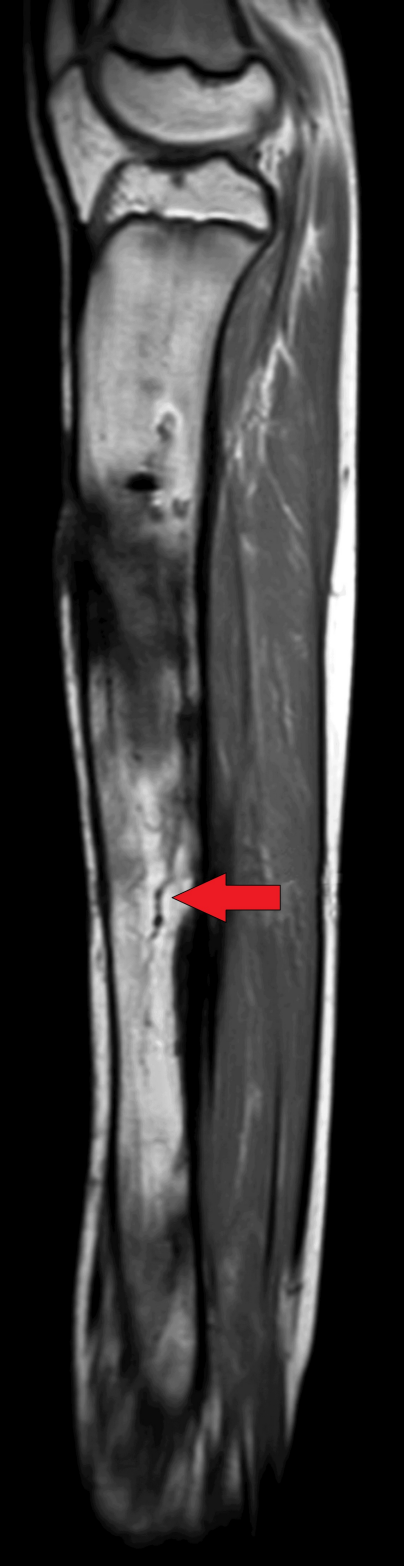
Sagittal T1WI MRI of tibia showing linear hypointense irregularities in the tibial diaphysis suggestive of areas of cortical erosion T1W1: T1-weighted imaging
